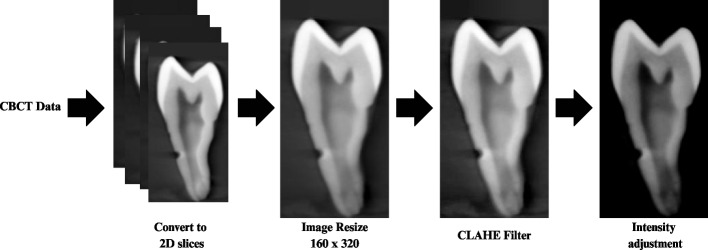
CBCT data image processing
